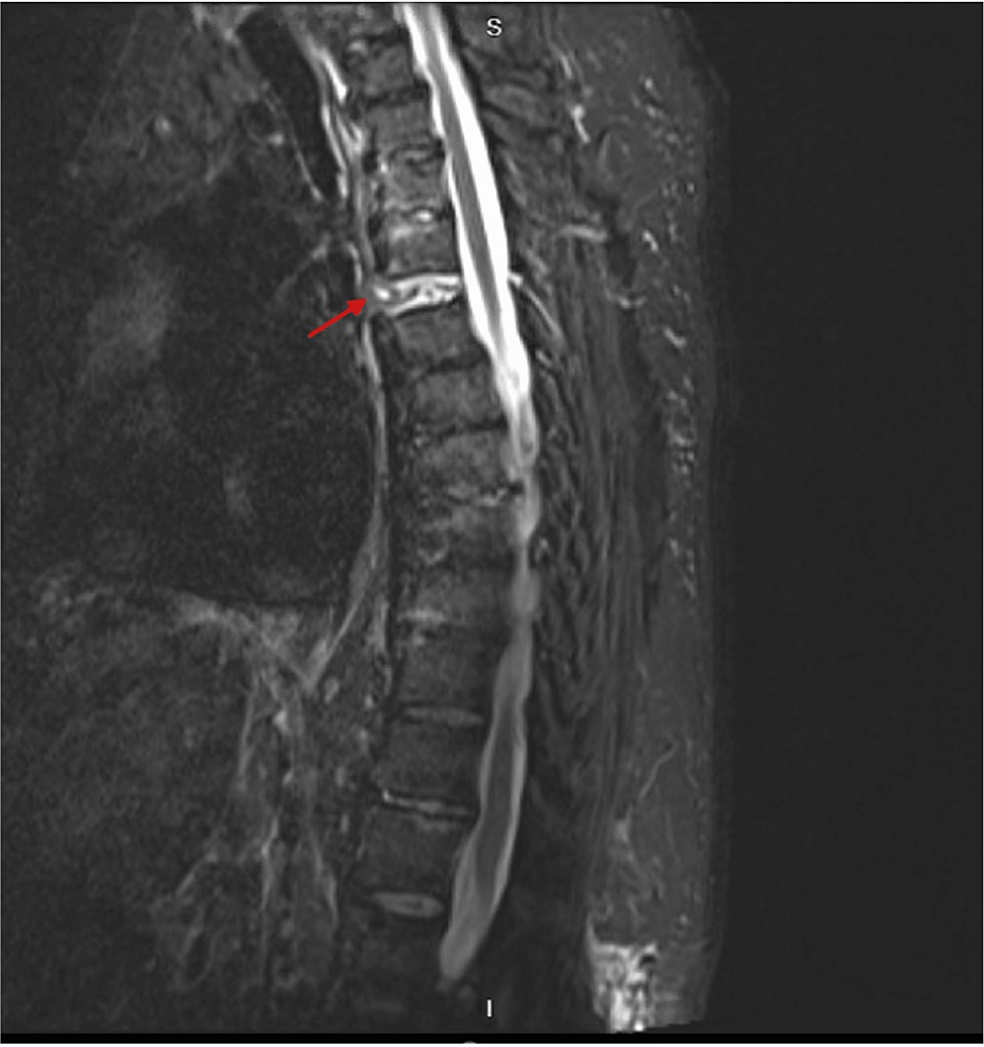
T2-STIR sagittal MRI Esophageal herniation into T4/5 disrupted disc space as indicated by arrow. STIR: short T1 inversion recovery
